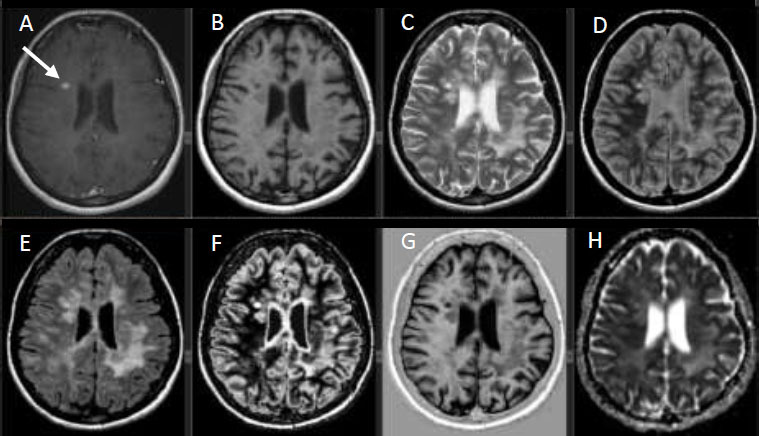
The registration of T1 post-contrast images with ADC and synthetic MRI scan, (A) Axial T1 post-contrast image, (B) Axial T1 pre-contrast image, (C) Synthetic Axial T2 image, (D) Synthetic PD image, (E) Synthetic FLAIR image, (F) Synthetic double inversion recovery (DIR) image, (G) Synthetic phase-sensitive image and (H) ADC image. The white arrow points to the enhancing MS lesion.
